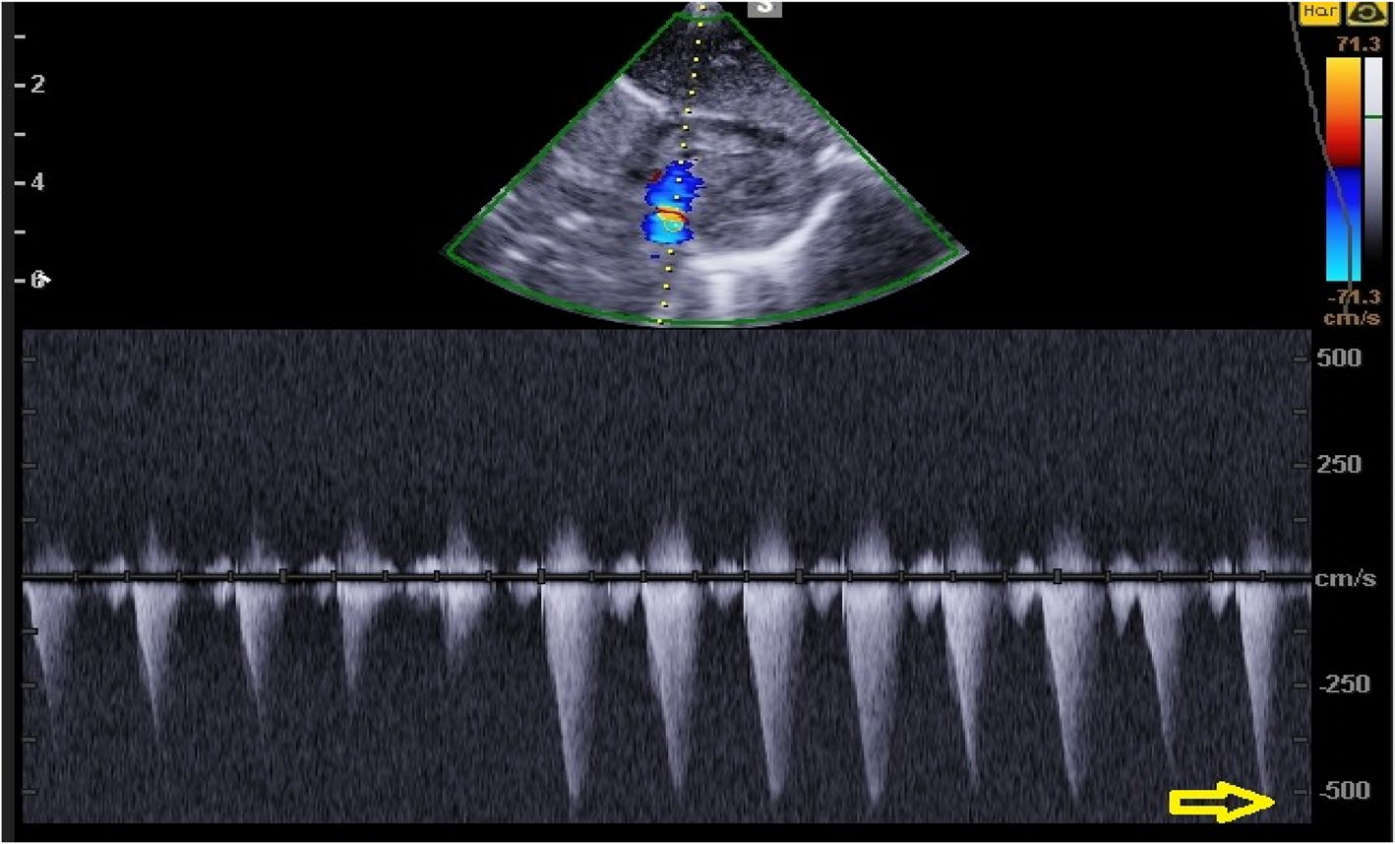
Doppler spectral in transthoracic echocardiography shows severe pulmonary valve stenosis early after birth (peak pressure gradient more than 100 mmHg)
